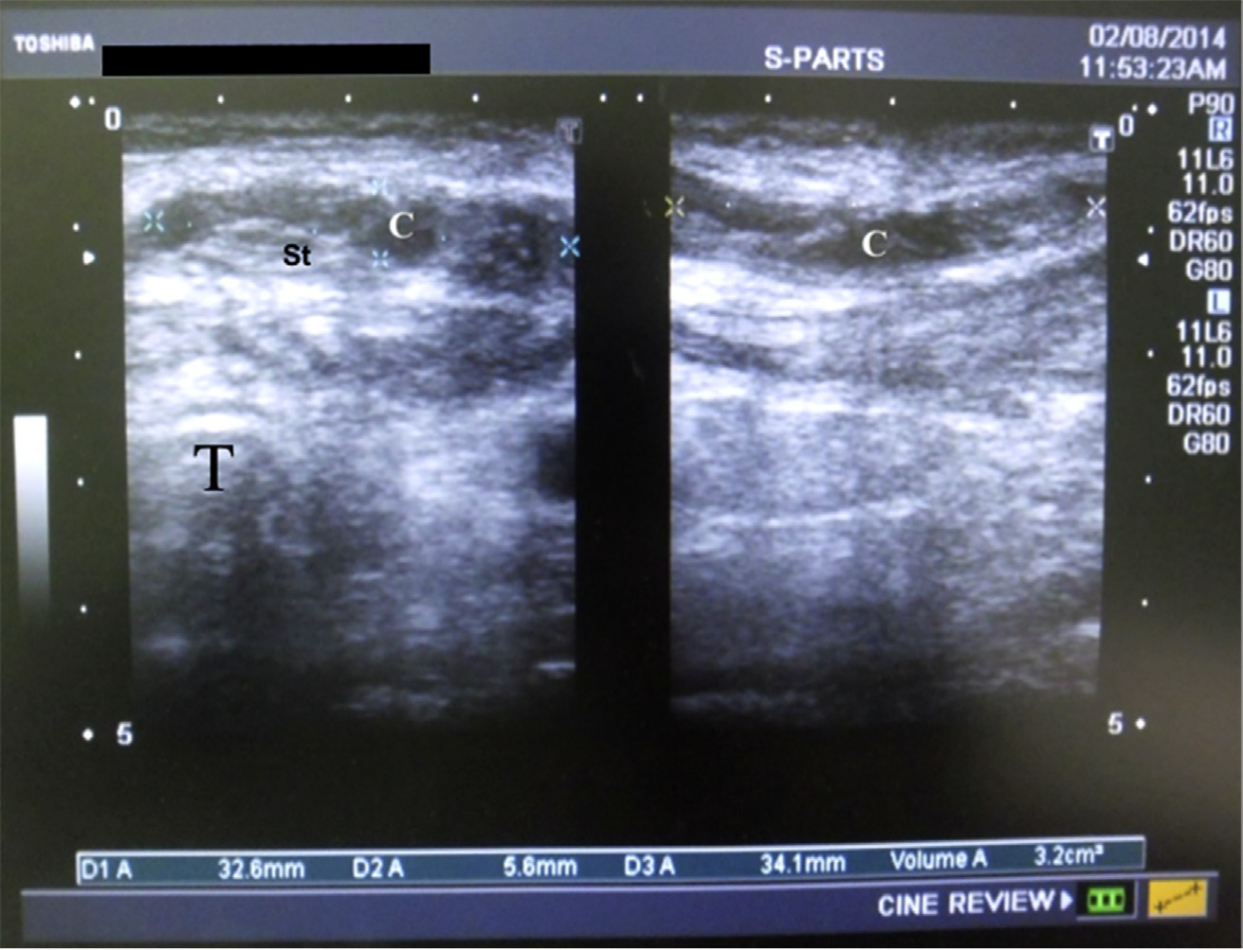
Ultrasound image of the neck with subcutaneous collection 2 days postoperative. Left side shows cross-section, right side shows saggital section.
